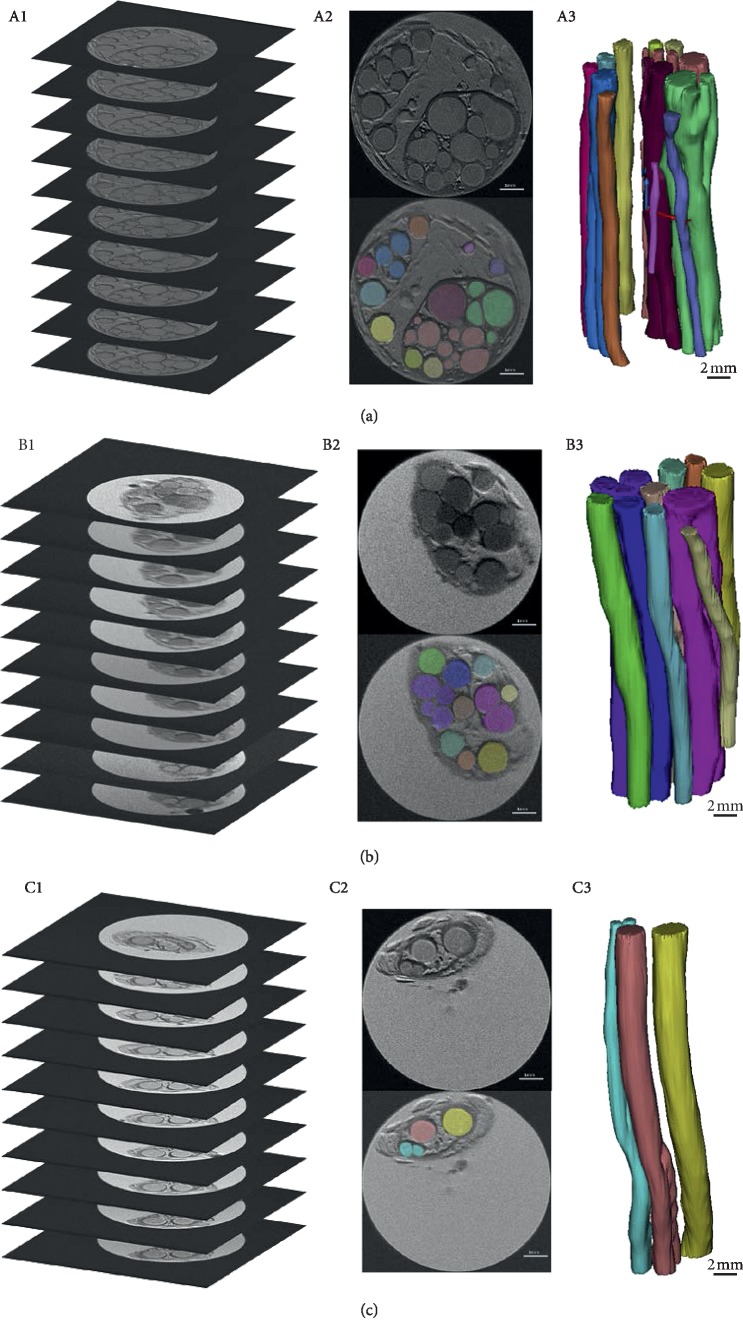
Micro-MRI scanning of the sciatic, tibial, and common peroneal nerves and three-dimensional reconstruction of the nerve fascicles. (a) Sciatic nerve Micro-MRI scan image (A1), two-dimensional image segmentation (A2), and three-dimensional reconstruction of nerve fascicles (A3). (b) Tibial nerve Micro-MRI scan image (B1), two-dimensional image segmentation (B2), and three-dimensional reconstruction of nerve fascicles (B3). (c) Common peroneal nerve Micro-MRI scan image (C1), two-dimensional image segmentation (C2), and three-dimensional reconstruction of nerve fascicles (C3). A2, B2, and C2: scale bar 1 mm; A3, B3, and C3: scale bar 2 mm.
